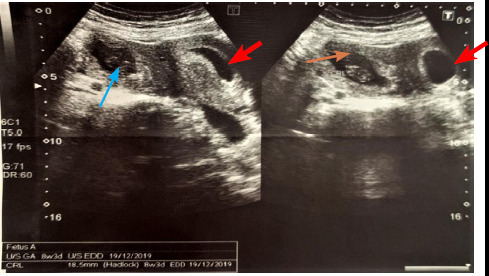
Transabdominal ultrasonography showing intrauterine gestation sac (red arrow) and right adnexal ectopic pregnancy with the single yolk sac (blue arrow) and single embryonic pole of 18.5 mm without cardiac activity (orange arrow).
